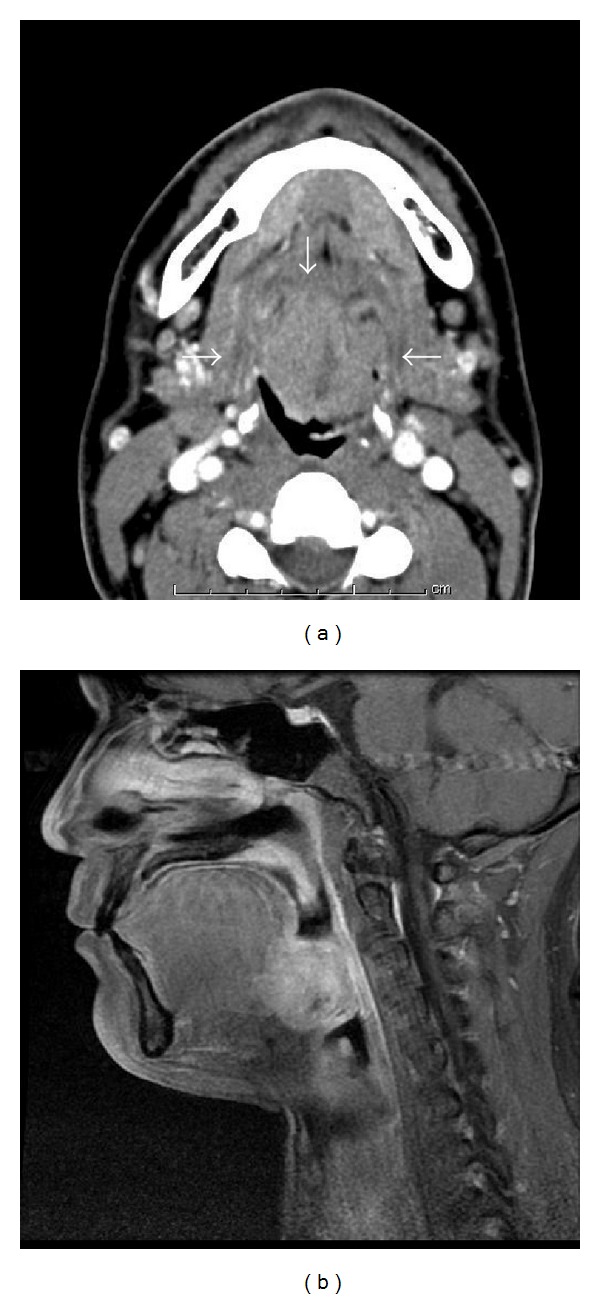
(a) An invasive tumor with a flat and smooth surface is seen at the midline of the base of the tongue (axial view of CT). (b) Enhanced MRI reveals a well-contrasted tumor, while the lower portion of the oropharynx is nearly closed (sagittal view of MRI).
